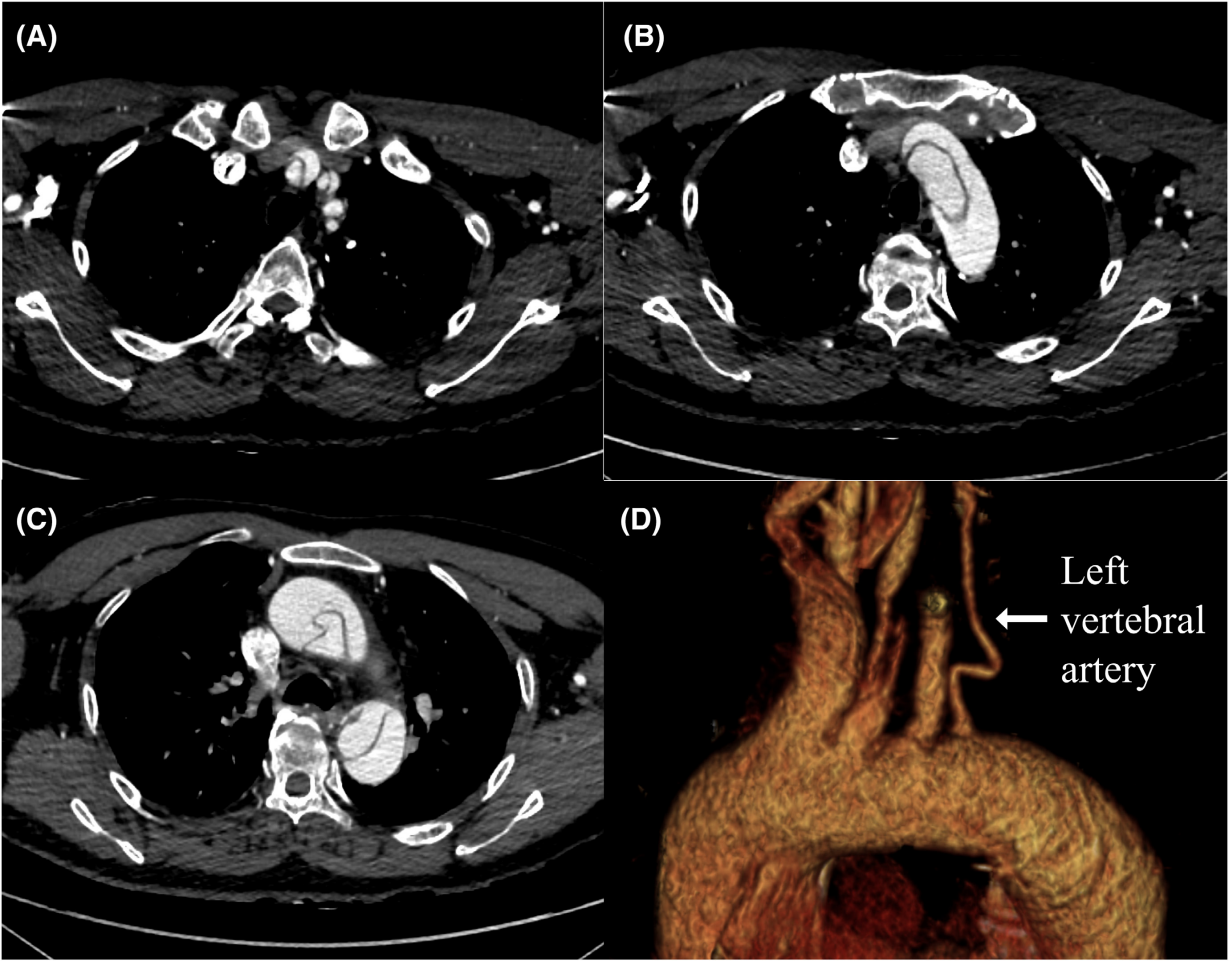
(A,B,C) Preoperative computed tomography showing a Stanford type‐A aortic dissection with an entry in the aortic arch. (d) Preoperative computed tomography showing an isolated left vertebral artery distal to the left subclavian artery
